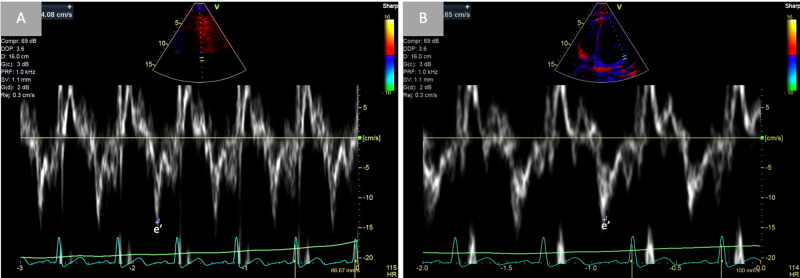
Annulus reversus Tissue Doppler imaging of the medial (A) and lateral (B) mitral annulus. In constrictive pericarditis, tethering of the lateral mitral annulus to the thickened pericardium leads a decrease in diastolic velocity (e’) over the lateral mitral annulus. Concomitantly, the medial e’ progressively increases as the severity of constriction worsens, leading to the characteristic finding of “annulus reversus”.
